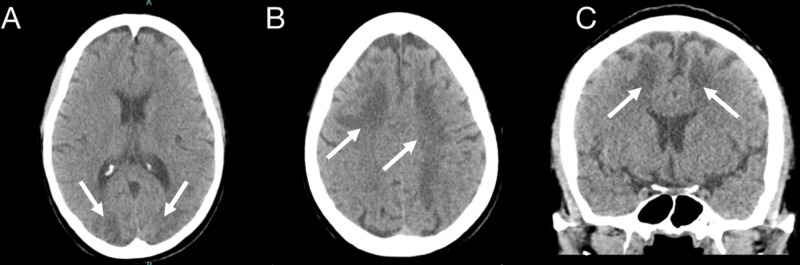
CT of the head without intravenous contrast: axial (A, B) and coronal (D) views Hypodense areas in the subcortical regions of the bilateral cerebral hemispheres involving the frontal, parietal and occipital lobes (arrows)
